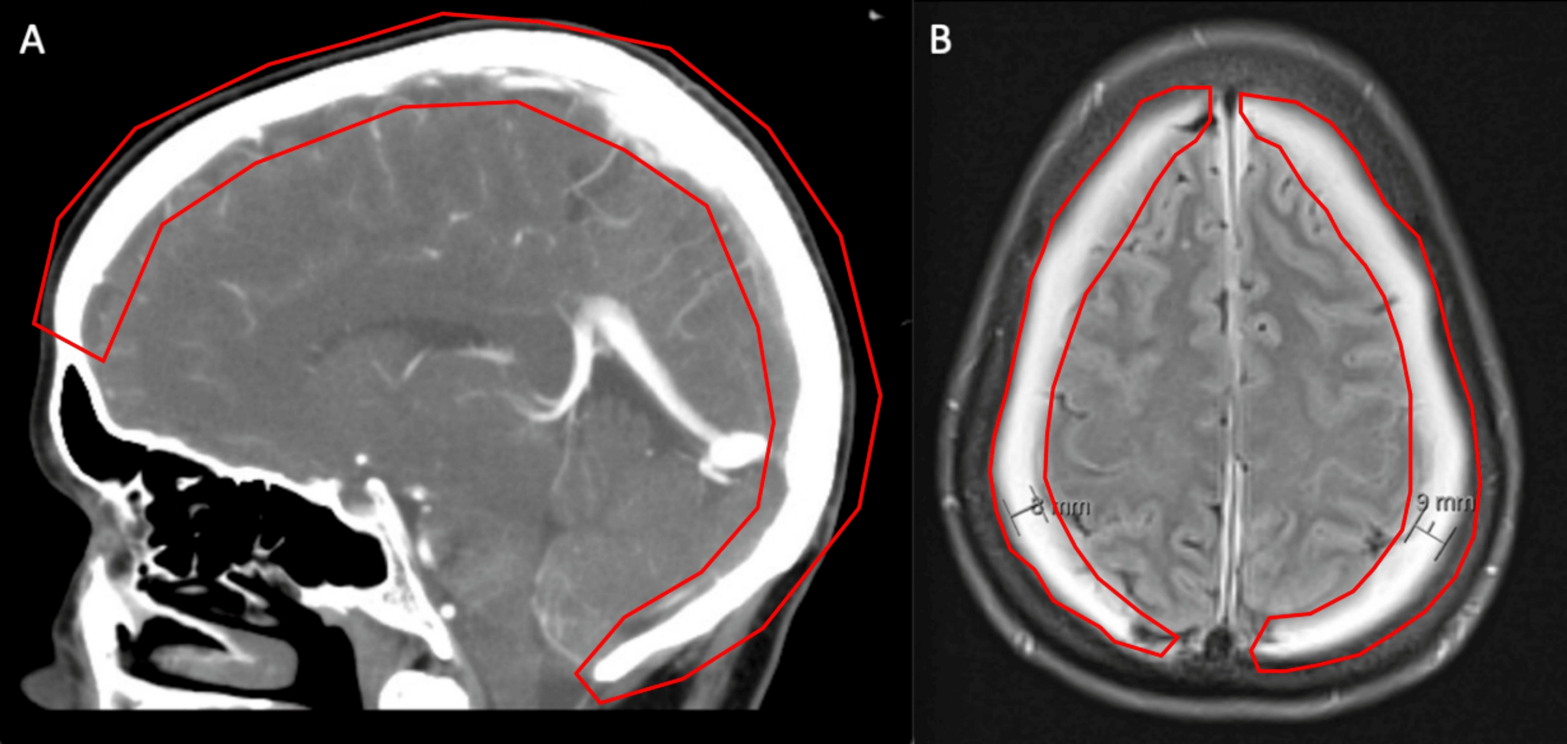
Sagittal CT and MRI scan emphasizing subdural hemorrhage. A) Sagittal contrast-enhanced CT view highlighting holo-hemispheric collections (red). B) Axial T2-weighted MRI showcasing bilateral holo-hemispheric collections and bilateral subdural hemorrhage (red). The sizes of the collections were 8 mm on the right and 9 mm on the left.
